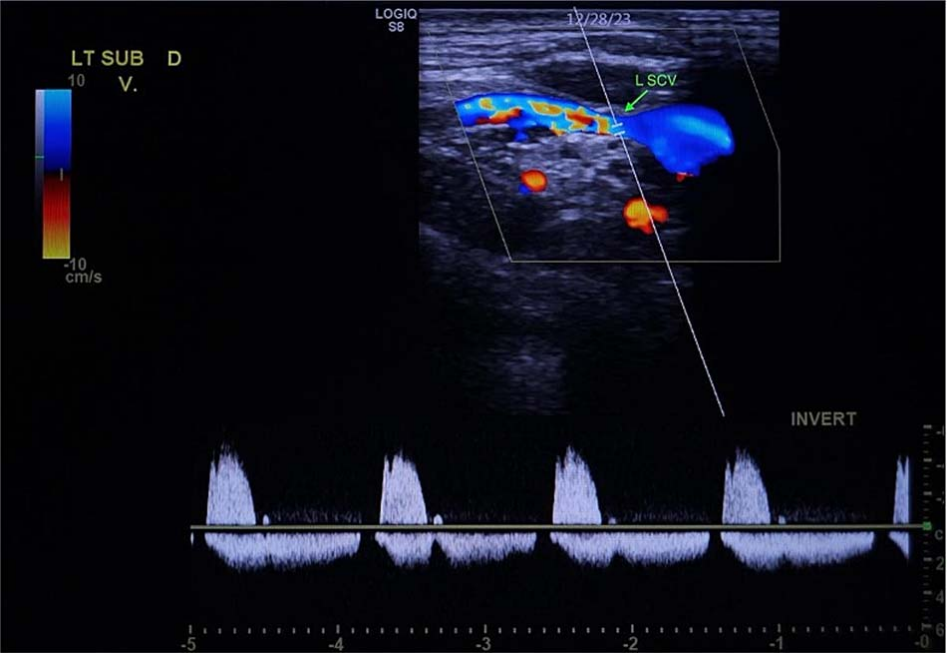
Demonstration of arterial waveform in the left subclavian vein on Duplex ultrasound (green arrow: left subclavian vein).
